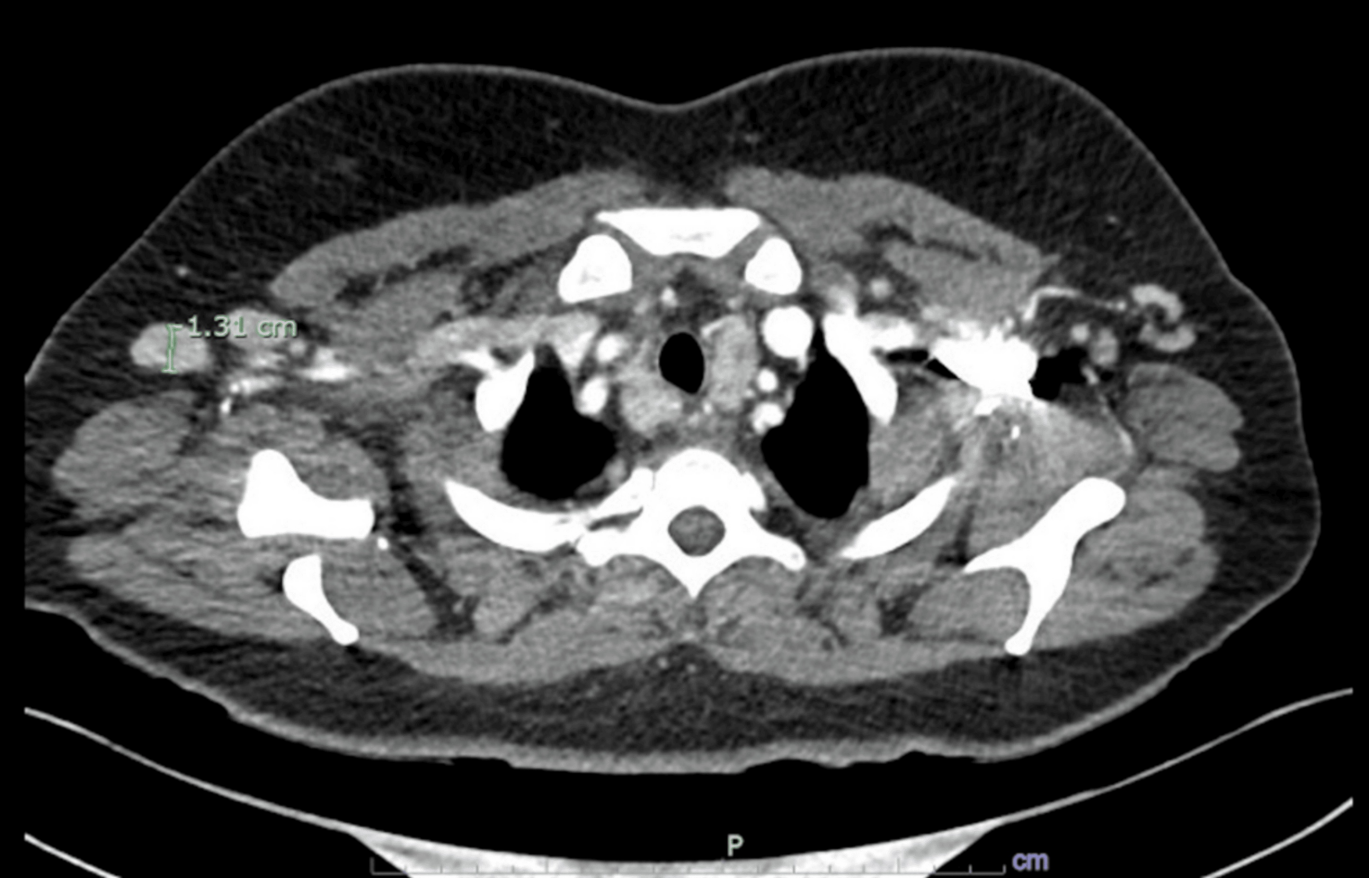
CT chest with contrast showing enlarged bilateral axillary lymph nodes measuring up to 1.31 cm in the short axis on the right (marked), as well as an increased number of subcentimeter mediastinal lymph nodes without pathologic enlargement.
